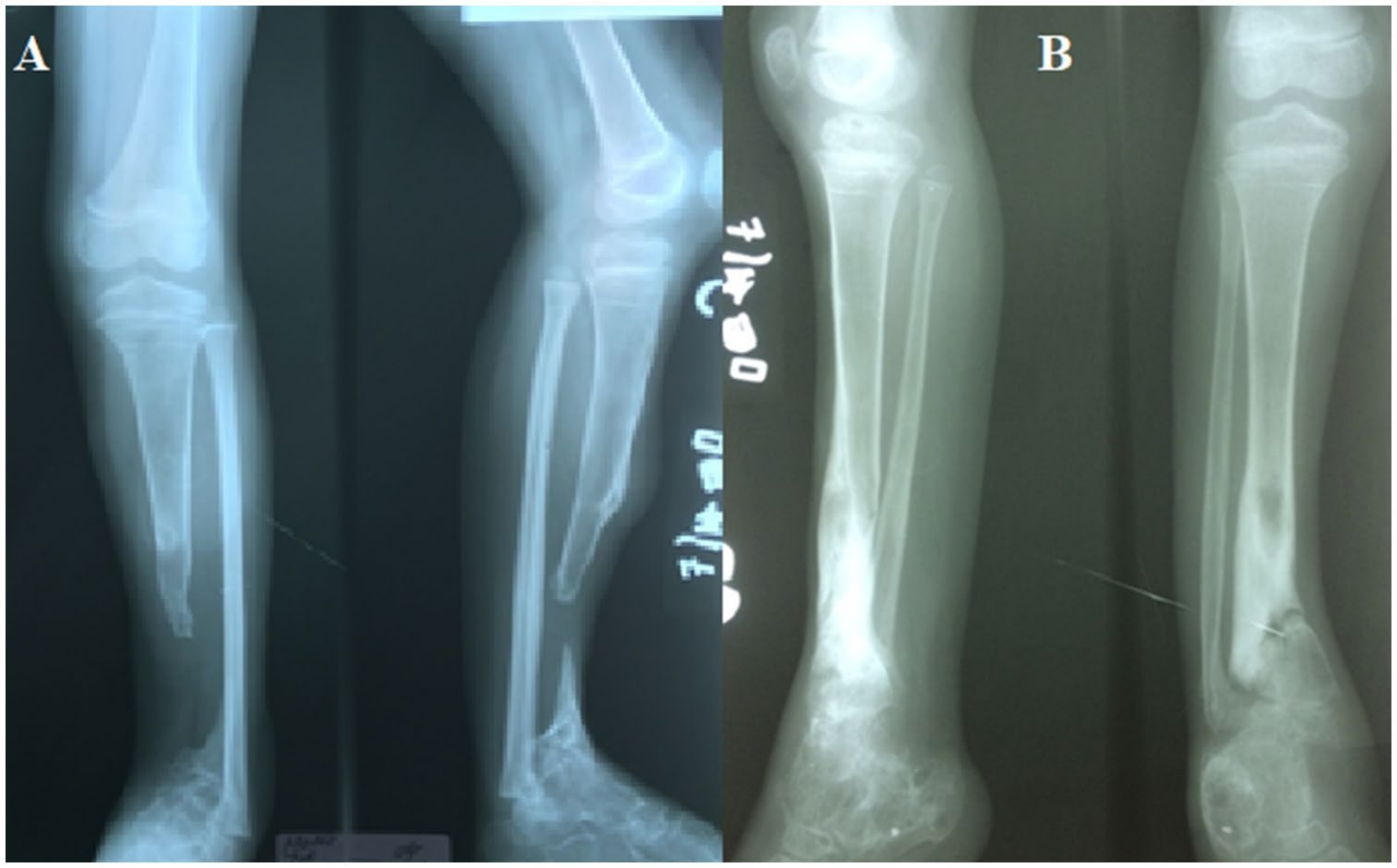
X-rays of both lower legs in 2 planes (A left, B right) 1 year after an open fracture of both lower legs after external fixation and a plaster of Paris
